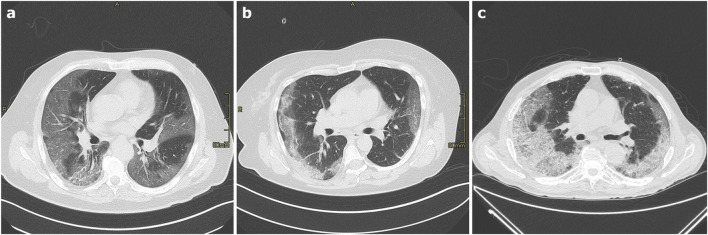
a–c Axial HRCT images of three different patients with COVID-19 pneumonia (a–c) showing bilateral, patchy ground-glass parenchymal opacities with prevalent peripheral and mid-lower lobes distribution
